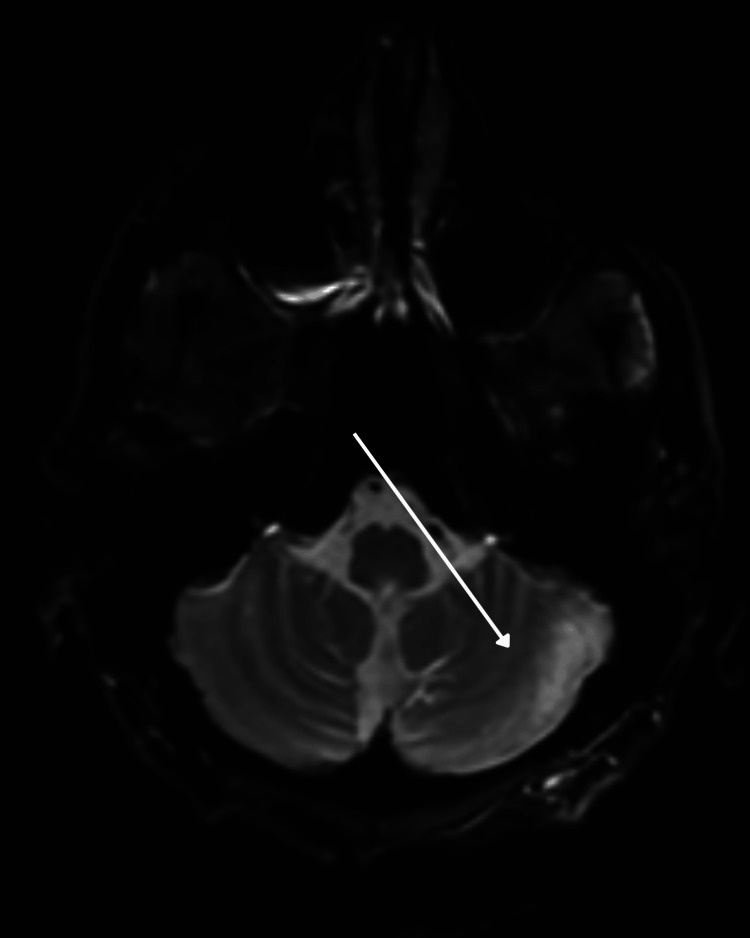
Axial T2/FLAIR MRI showing extensive bilateral cerebral white matter hyperintensity with patchy involvement of the anterior and middle cerebellar peduncles, more prominent on the right (arrow). FLAIR: fluid-attenuated inversion recovery
